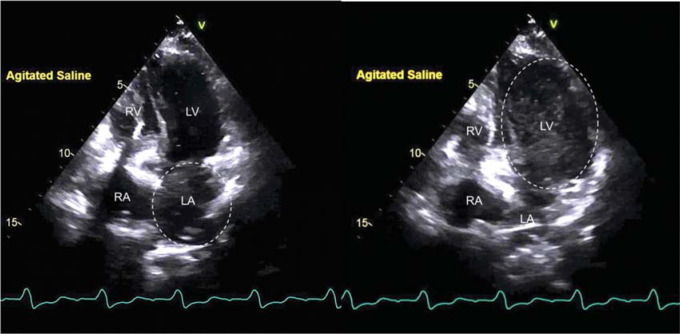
Apical 4-chamber transthoracic echocardiogram showing how agitated saline contrast bubbles initially appeared in the LA (as shown by the dashed circle) on the left image, followed by the left ventricle (shown by the dashed oval) on the right image. In normal anatomy, the bubbles will appear in the right atrium first, followed by the right ventricle right after injection. *Abbreviations:* RA, right atrium; RV, right ventricle.
